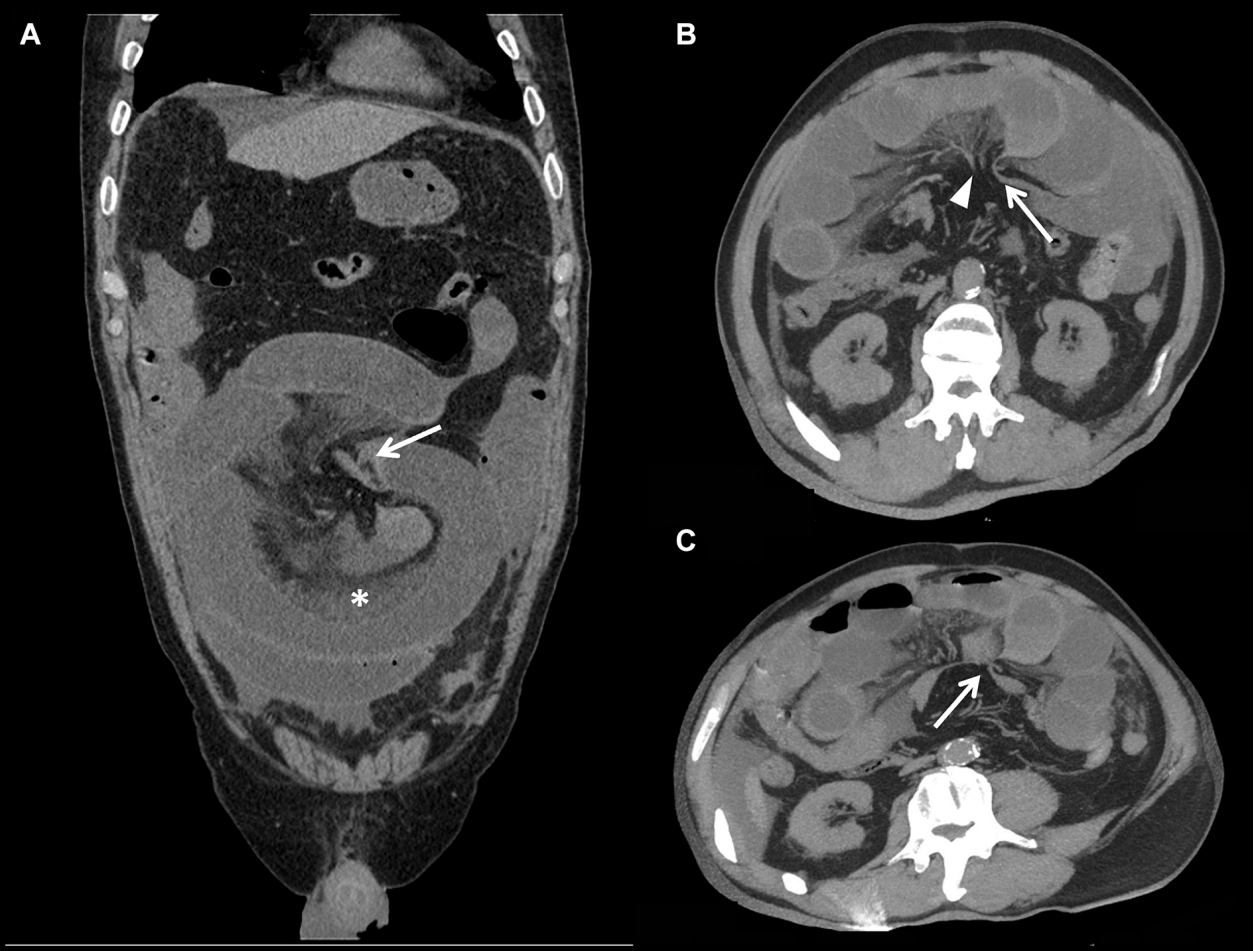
Non-enhanced CT images in 59-year-old man show strangulated ileus, transmural necrosis of the small bowl wall due to internal hernia. Panel (A) is MPR (multi-planar reconstruction) image. Panels (B,C) are MIP (Maximum intensity projection) images reconstructed from different angles. The images show that the small intestine herniated into the greater omentum is dilated with fluid accumulation, and the attenuation of the intestinal wall is increased. The white arrow points to the site of intestinal strangulation. Mesenteric vessels are interrupted at the site of strangulation (arrowhead), and proximal mesenteric vessels are tortuous and dilated. Simultaneously, the mesenteric haziness (*) was shown.
